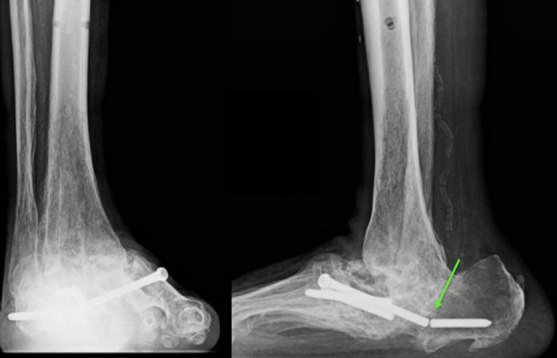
Case 3 mortis and lateral ankle radiographs showing tibiocalcaneal nonunion with a broken screw (arrow).
